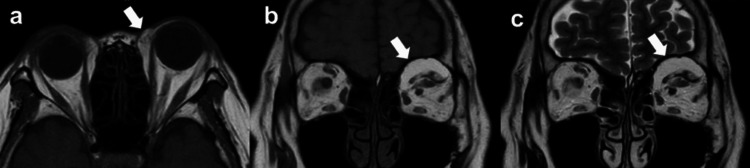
MRI images taken at the first visit to the previous hospital. a, b. Axial and coronal T1-weighted MRI images showing a homogeneous, high-intensity mass involving the superior oblique muscle in the superior orbit (arrow); c. Coronal T2-weighted MRI image showing a high-intensity mass (arrow).
